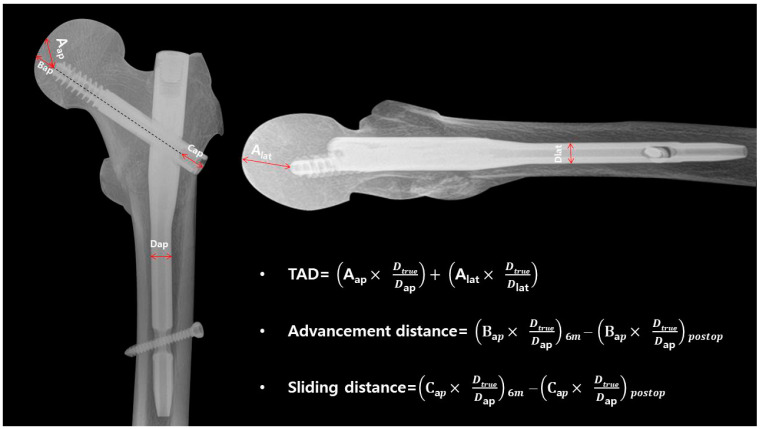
In the AP view of the X-ray for A_ap_, the distance was measured from the lag screw tip to the apex of the femoral head. For B_ap_, the measurement was taken from the lag screw tip along the axis to the point where it intersects with the femoral head. C_ap_ represents the distance from the nail to the lag screw end, and D_ap_ represents the measurement of the nail’s distal diameter. In the lateral view X-ray, A_lat_ represents the distance from the lag screw tip to the apex of the femoral head, while D_lat_ signifies the measurement of the nail’s distal diameter. All measurements were corrected for magnification using the actual length of the diameter.
